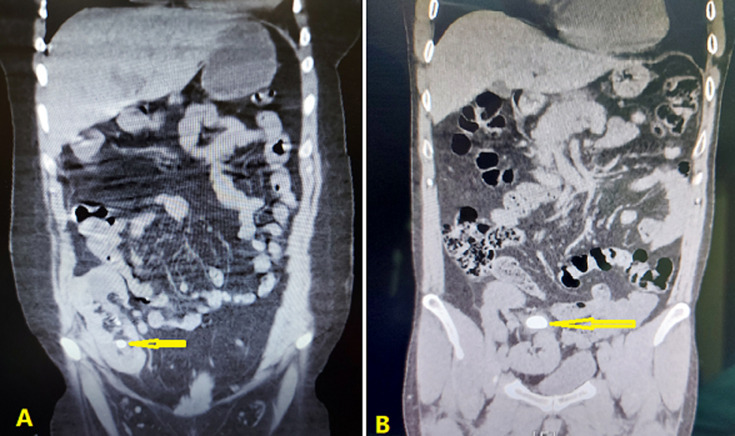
preoperative abdominal pelvic computed tomography (CT) scan showing: A) the lower pole renal stone in the first case (arrow); B) the stone in the renal pelvis in the second case (arrow)
